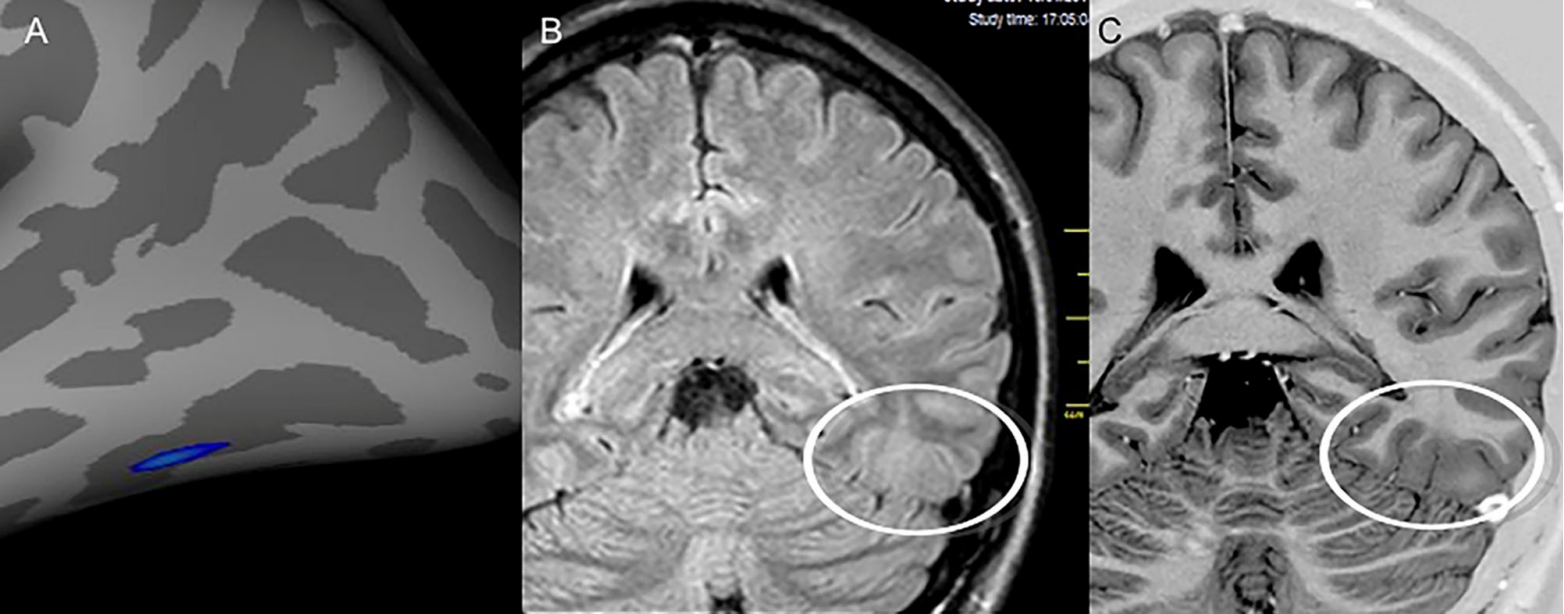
Images from patient 24 who was initially classified as magnetic resonance imaging (MRI) negative. After visual evaluation guided by postprocessing, a potentially epileptogenic structural lesion was identified in the same region considered the epileptogenic zone by electroclinical data. A) Results of the postprocessing of cortical thickness in the FreeSurfer software environment, showing cortical thickening in the left inferior temporooccipital transition. Nonvolumetric coronal fluid attenuation inversion recovery (FLAIR) (B) and T2 short tau inversion recovery (STIR) with inverted window (C) acquisitions showing discrete focal cortical thickening and white–gray transition blurring (white circles) in the same region identified by the quantitative analysis.
